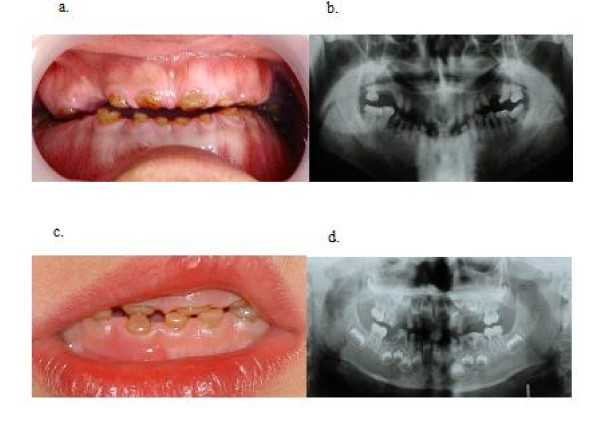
Clinical analysis of dentinogenesis imperfecta type II (DGI-II). The proband (IV5) is a man aged 32. His permanent teeth showed a shade of brown and almost complete attrition of the enamel layer without a history of periapical infections(a and b). Dentition of the 5-year-old son of the proband. His primary teeth had shown normal timing of eruption, but shortly thereafter become brownish and small due to cracking of the enamel and attrition of dentin. At the time of examination, his first permanent molars had just emerged and still showed an intact enamel(c and d).
